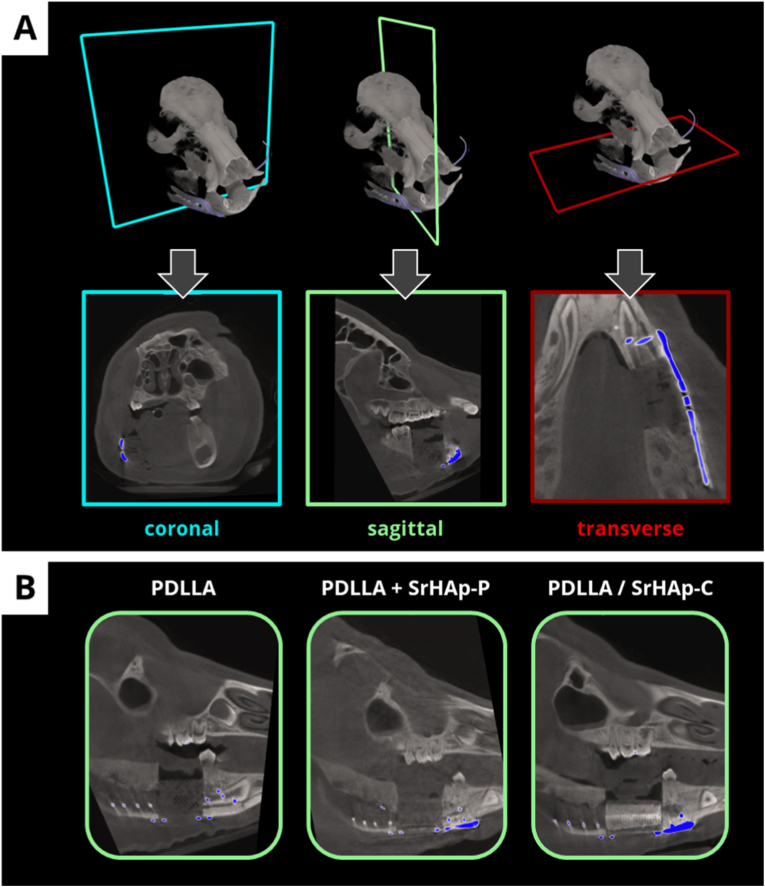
DVT measurement after implantation. (A) Location of the coronal (blue), sagittal (green), and transverse (red) plane in the 3D reconstruction as well as (B) radiographic appearance of the three implant variants immediately after implantation in sagittal view.
